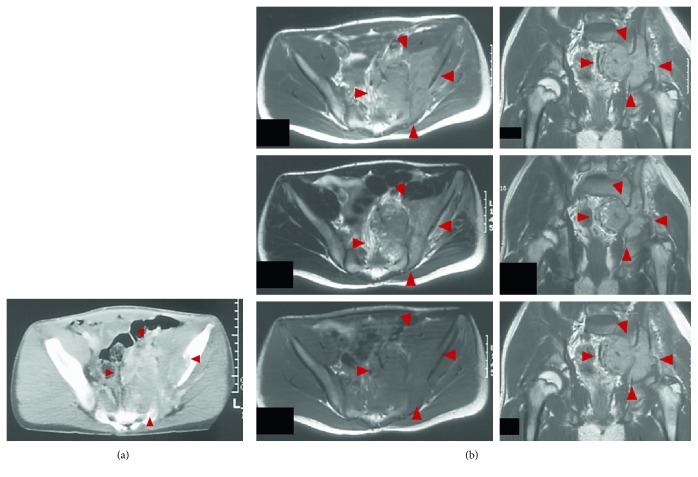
(a) 7.2 × 9.5 × 3.0 cm mass arising from the left iliac bone and extended into the sacral bone. (b) The large mass showing low intensity on T1-weighted images and high intensity on T2-weighted images and sparsely enhanced by contrast agent.
